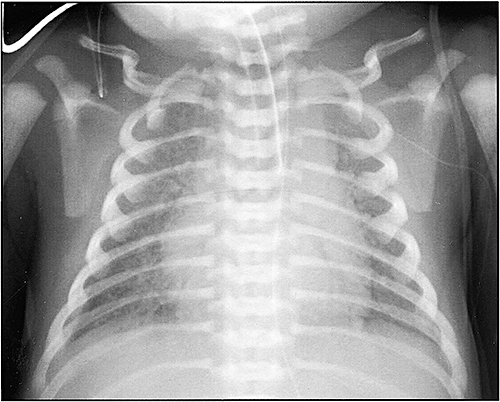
Plain chest X-ray. A chest X-ray film taken just before the patient's death shows marked pulmonary congestion and diffuse bilateral reticulogranular infiltrates. No apparent cardiomegaly is seen.
